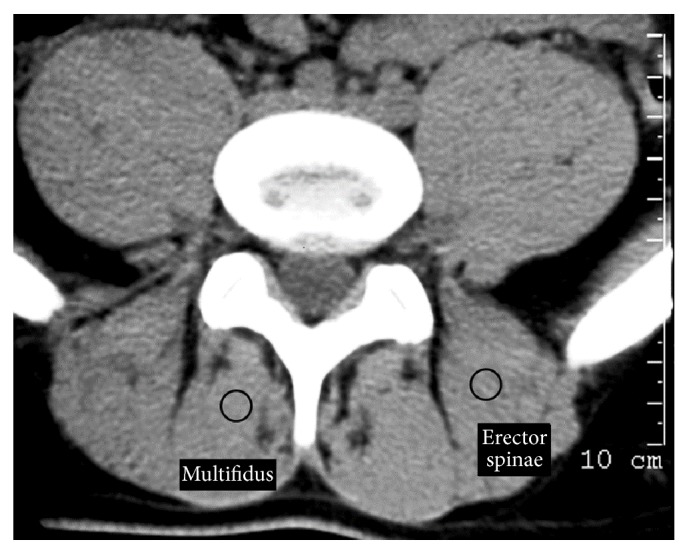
An example of a paraspinal muscle density evaluation using a 6 mm circle in the center of the most preserved muscle mass positioned on the noncontrast axial lumbar spine CT (L5-S1 spinal level) of a 34-year-old male subject.
